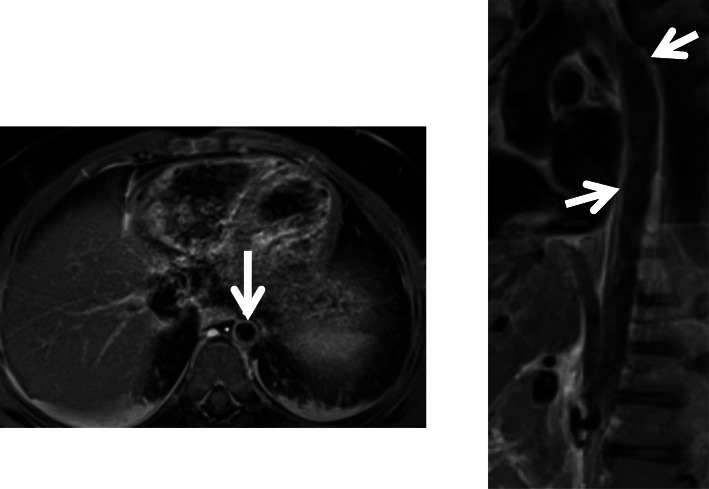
Magnetic Resonance Imaging Angiogram. a Axial view showing diffuse thickening and abnormal enhance of the thoracic and abdominal aortic walls indicative of diffuse aortitis (arrow) b, Sagittal long-axis view showing diffuse thickening (arrows) and abnormal enhance of the thoracic and abdominal aortic walls indicative of diffuse aortitis
